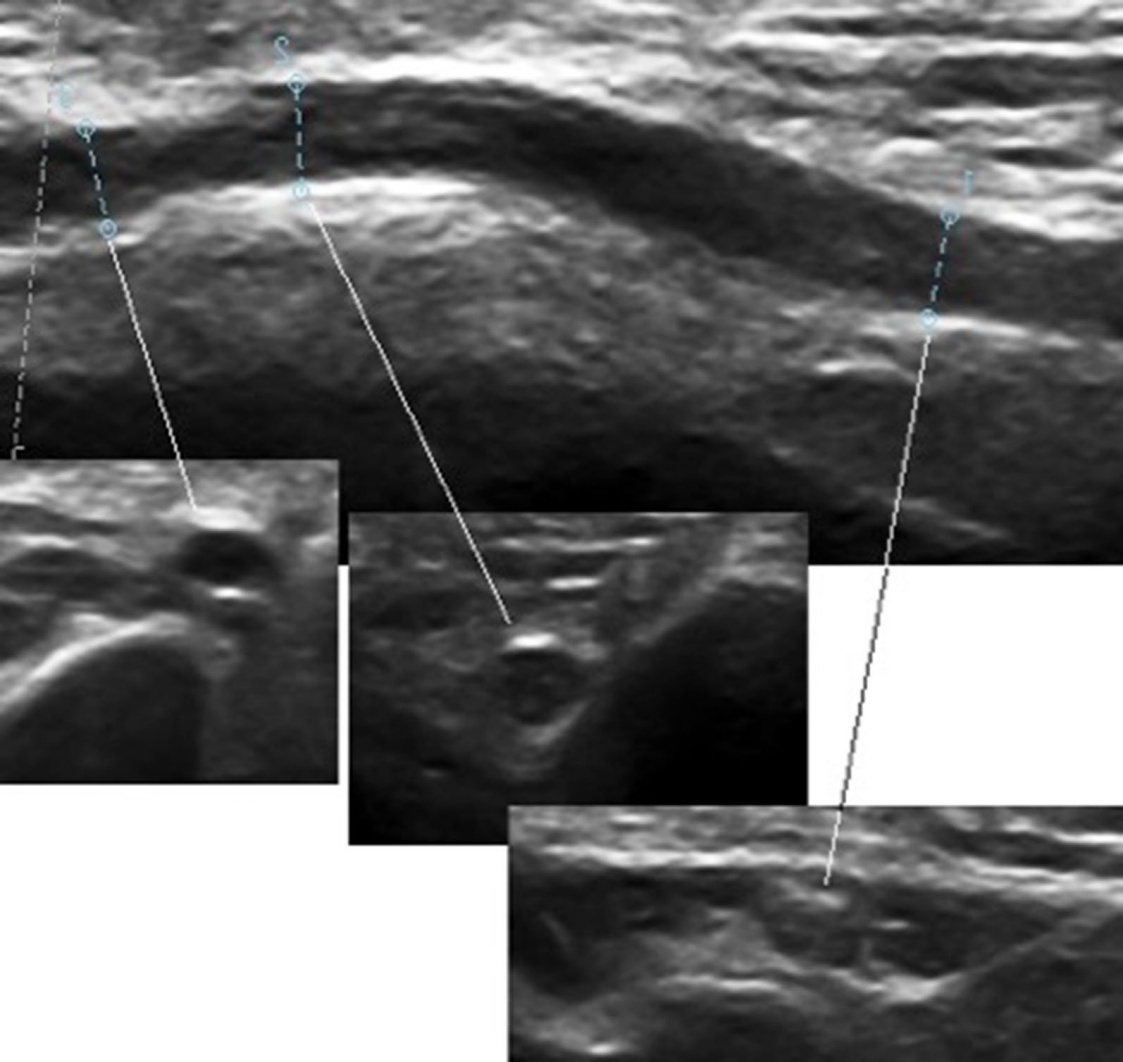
Above, horizontal elbow position. Ultrasonographic evalution of ulnar groove. Left proximal segment, right distal segment Below, transversal evaluations. Right, distal segment (ulnar tunnel); middle and left proximal segment (ulnar groove)
